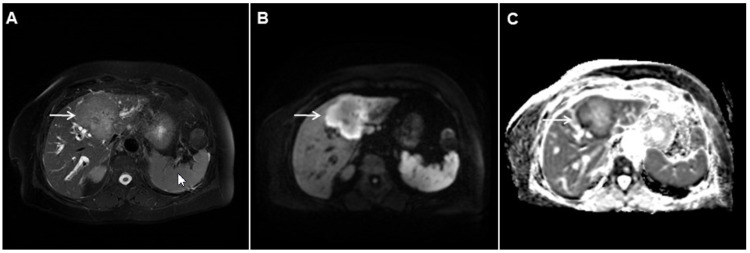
Mass-forming intrahepatic cholangiocarcinoma in the left liver lobe of a 76-year-old man. Axial T2-weighted FS image shows lobulated hetrogeneously hyperintense hepatic tumor (*arrow*) with perilesional biliary dilatation (A). Axial diffusion-weighted image (b = 800 s/mm^2^) shows target-like appearance (*arrow*) of the lesion that consists of a central darker area and a peripheral hyperintense area (B). Corresponding ADC map is shown on (C).
